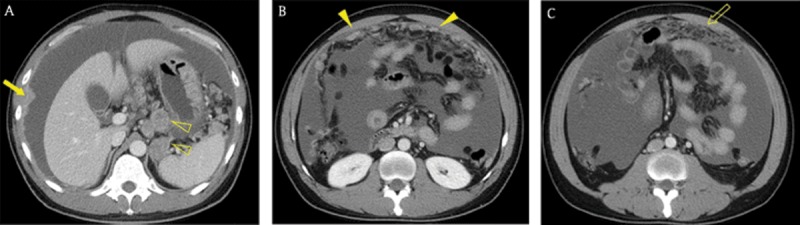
A 40-year-old man with advanced gastric cancer and peritoneal carcinomatosis. Axial portal venous phase CT images revealed multifocal discrete nodules (arrowheads) in the peritoneal cavity, peritoneal enhancement and thickening (arrow), ascites, and omental haziness (open arrow). Note the metastatic lymphadenopathy around the stomach (open arrowheads).
